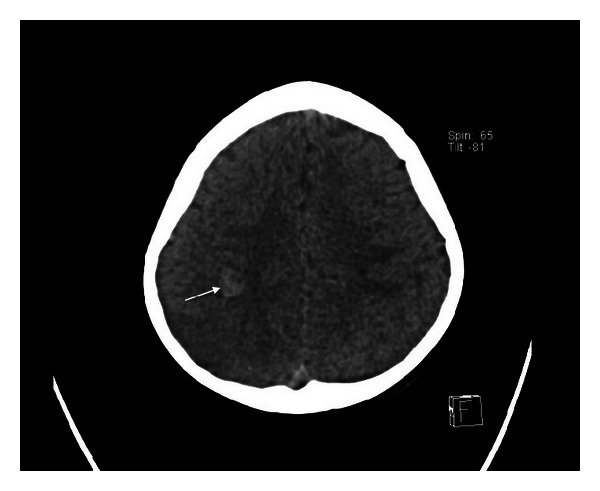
Plain CT scan demonstrated 10 mm × 15 mm right frontal intracerebral hemorrhage (arrow).
